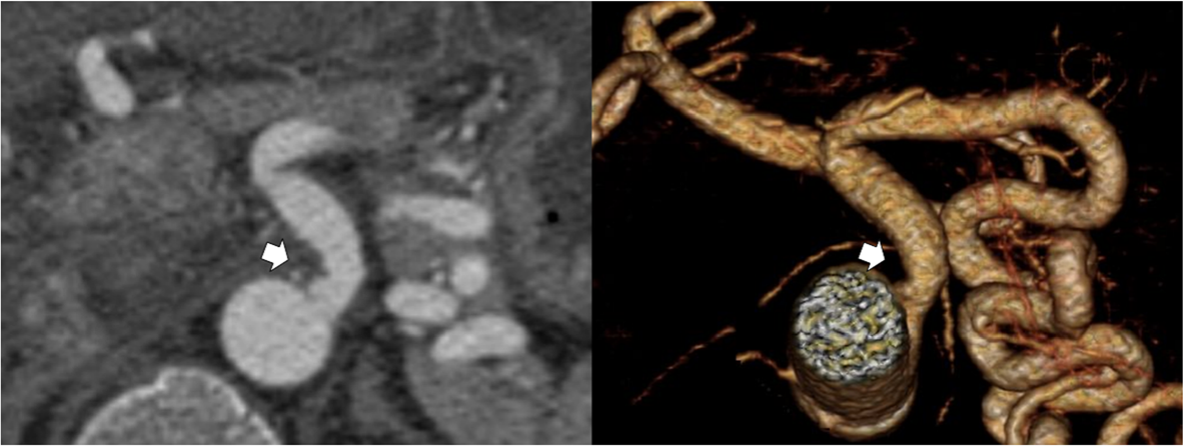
Contrast-enhanced computed tomography angiogram of the abdomen demonstrates a dissection of the celiac trunk (*arrows*). Axial (*left panel*) and three-dimensional (*right panel*) reconstruction images show an abrupt narrowing of the vessel in the absence of atherosclerotic plaque or extrinsic compression
